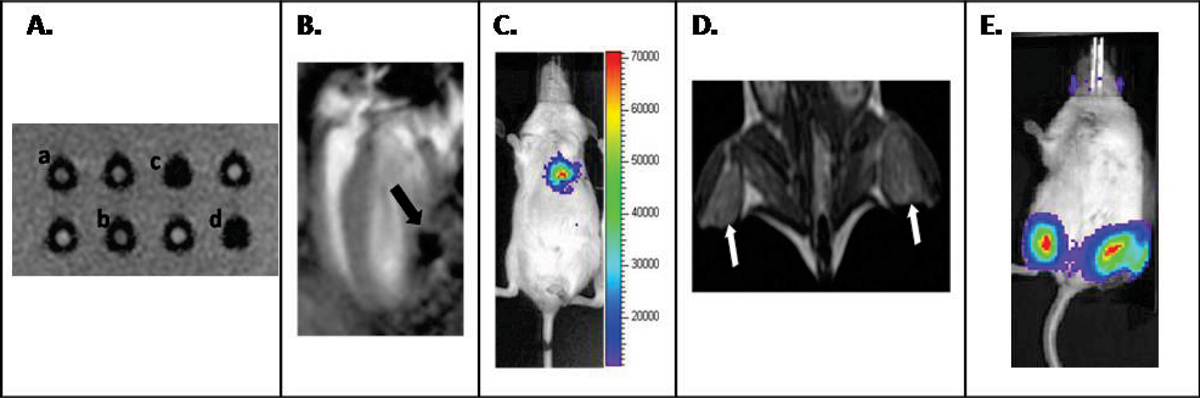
Functional confirmation of the MRI RG and MRI and BLI. A
